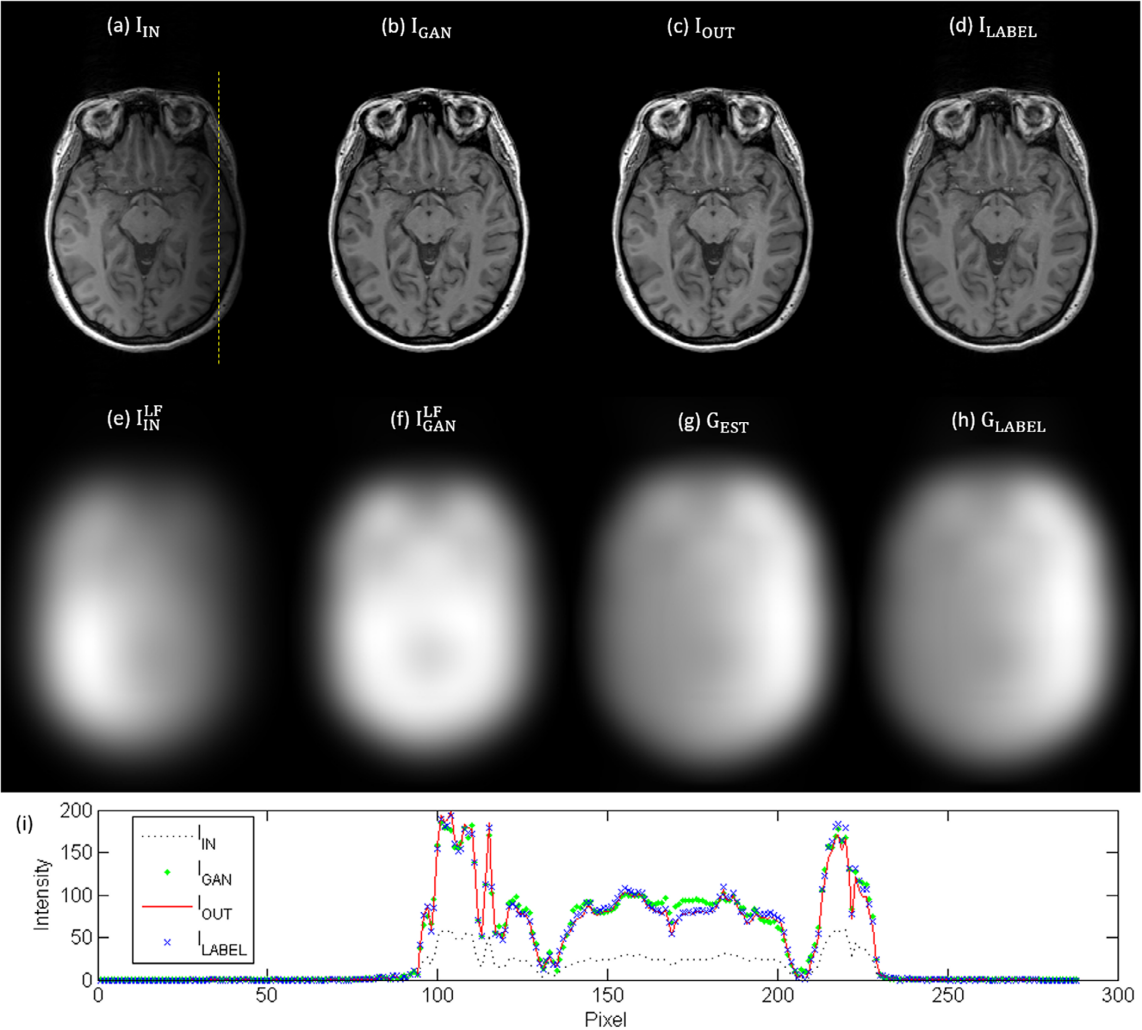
Example of (a) $I_{\mathrm{IN}}$, (b) $I_{\mathrm{GAN}}$, (c) $I_{\mathrm{OUT}}$, (d) $I_{\mathrm{LABEL}}$, (e) $I_{\mathrm{GAN}}^{\mathrm{LF}}$, (f) $I_{\mathrm{IN}}^{\mathrm{LF}}$, (g) $G_{\mathrm{EST}}$, (h) $G_{\mathrm{LABEL}}$ for T1‐weighted brain MR images and (i) intensity profiles along the yellow dashed line for $I_{\mathrm{IN}}$, $I_{\mathrm{GAN}}$, $I_{\mathrm{OUT}}$, $I_{\mathrm{LABEL}}$.
